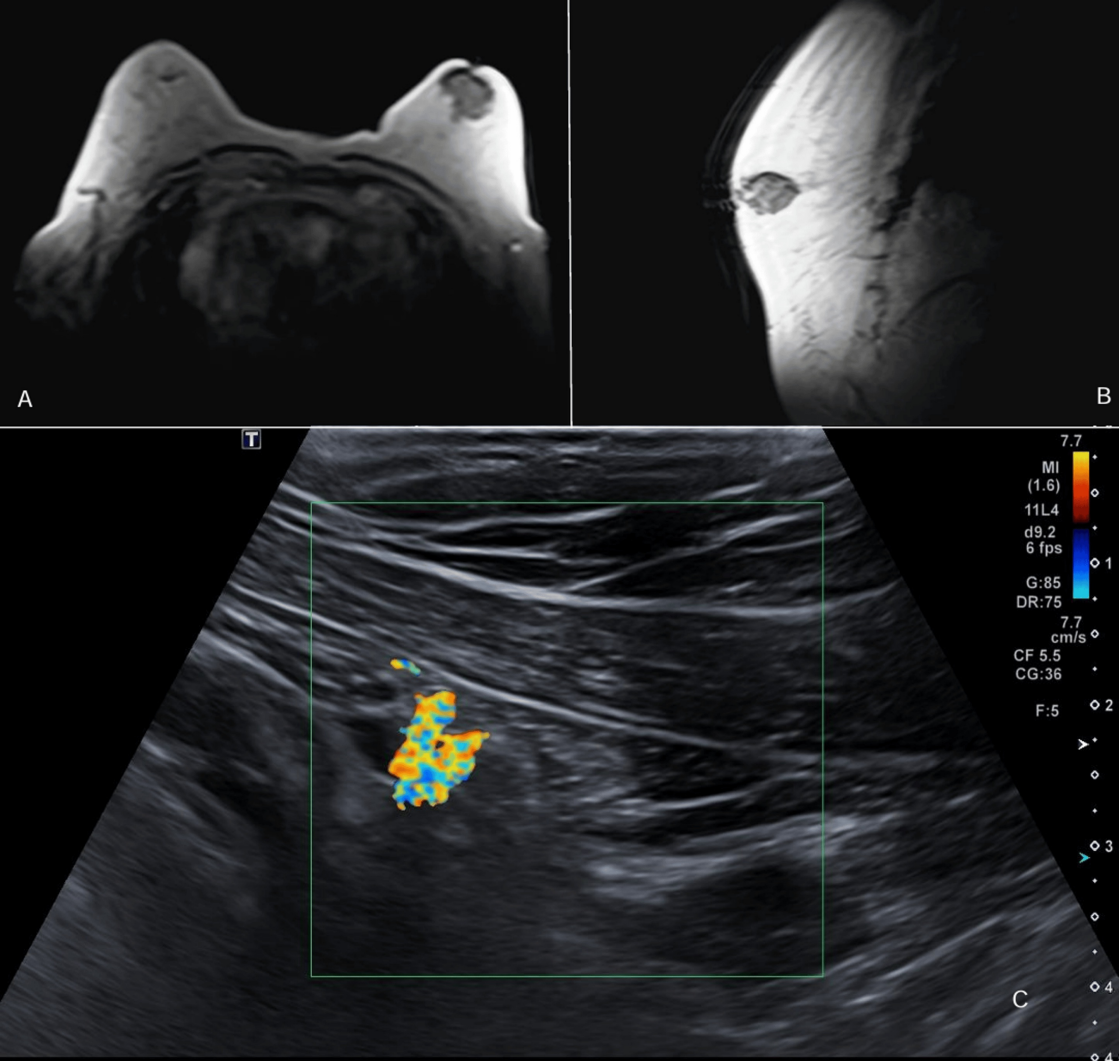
(A,B) Magnetic resonance imaging and (C) ultrasound of the breast (A,B) A hypodense lesion in the left breast suggestive of a nodule with irregular characteristics, angulated margins, and solid components. (C) The nodule measures approximately 40.4 × 26.3 × 43 mm, showing penetrating vascularity on color Doppler and minimal acoustic enhancement.
